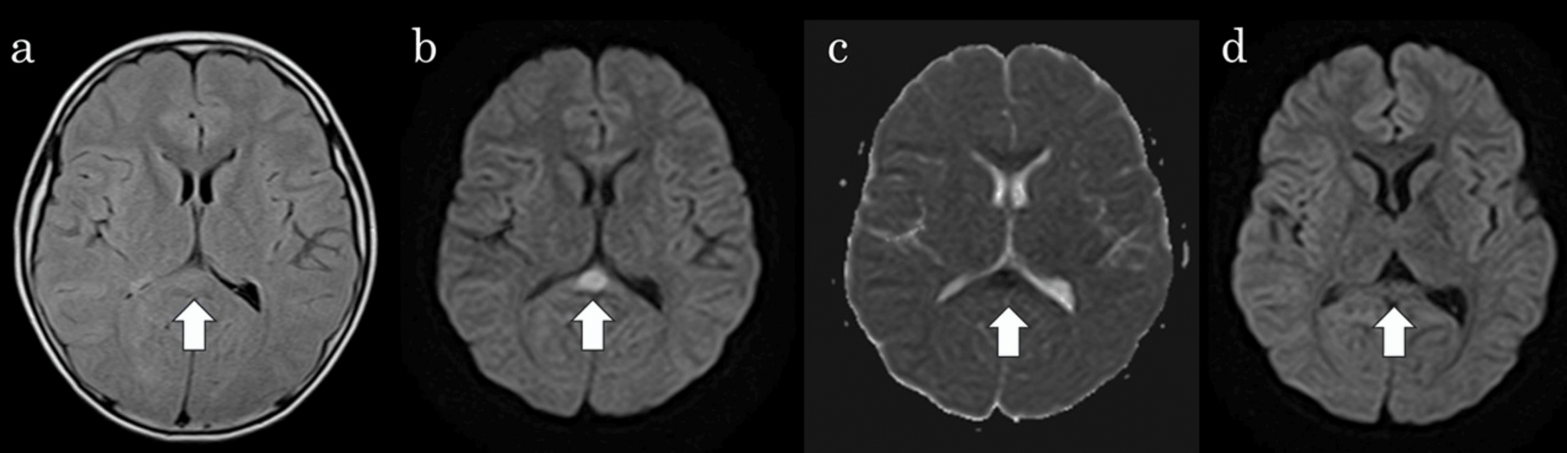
Brain MRI Initial MRI revealed abnormal signals in the splenium of the corpus callosum (arrow), which were of somewhat hyperintensity on fluid-attenuated inversion recovery imaging (a), hyperintensity on diffusion-weighted imaging (DWI) (b), and hypointensity on apparent diffusion coefficient (ADC) mapping (c). Follow-up MRI showing complete improvement of the SCC on DWI (arrow) (d). SCC: Splenium of the corpus callosum
